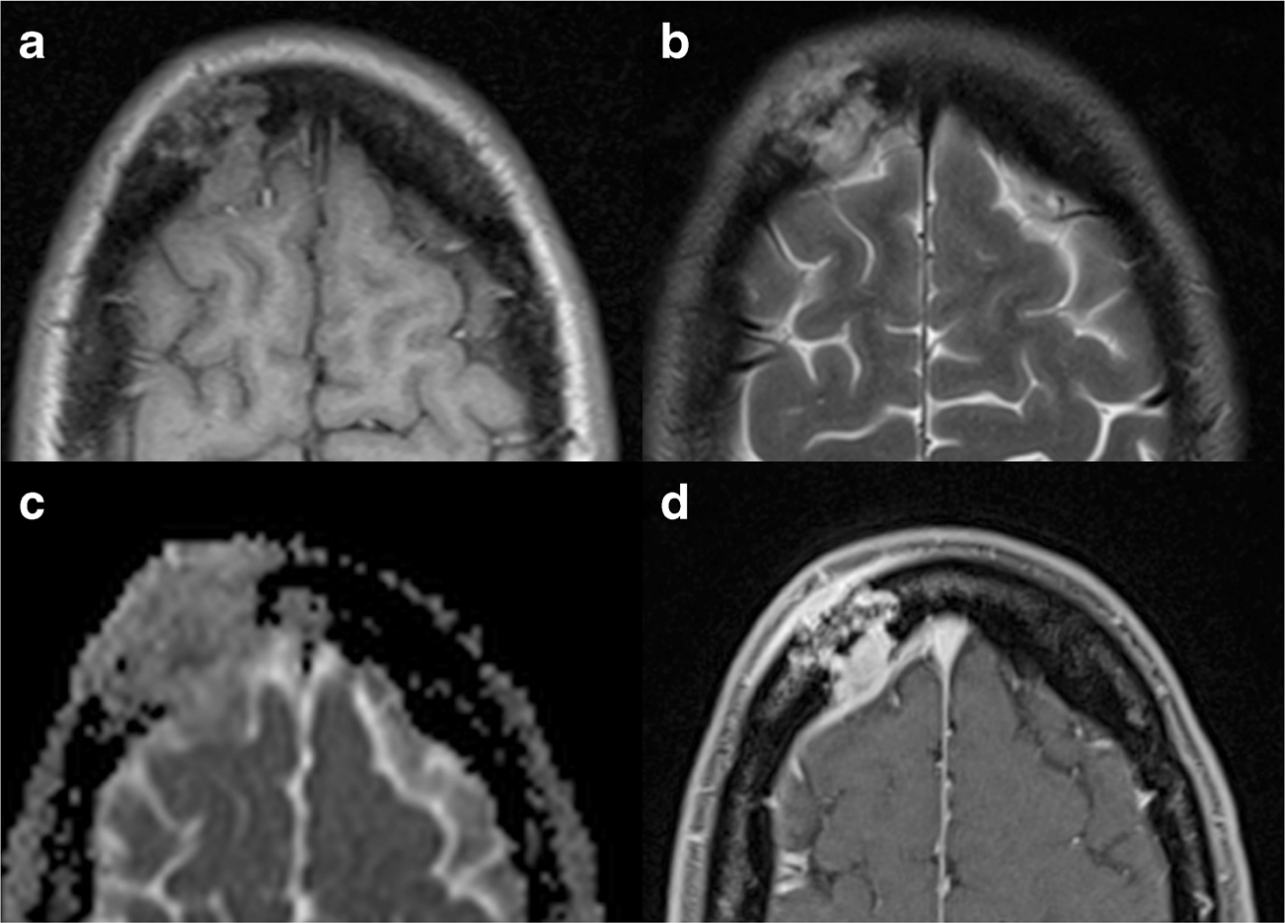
EG, same patient shown in Fig. 9. On T1w (a) and T2w (b) images, the lesion appears mildly inhomogeneous, with diffusion restriction on the ADC map (c). MRI allows for an accurate evaluation of the surrounding soft tissues, as well as intracranial extension after contrast administration (d), causing a moderate dural thickening
